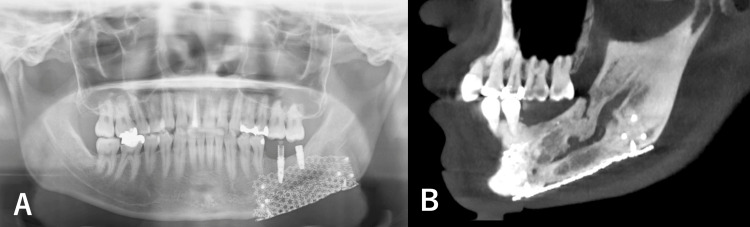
Postoperative radiograph findings A: Panoramic radiograph after the placement of dental implants. B: CBCT image. Postoperative radiographs show satisfactory bone regeneration and formation of a mandibular-like lumen. CBCT: Cone-beam CT
